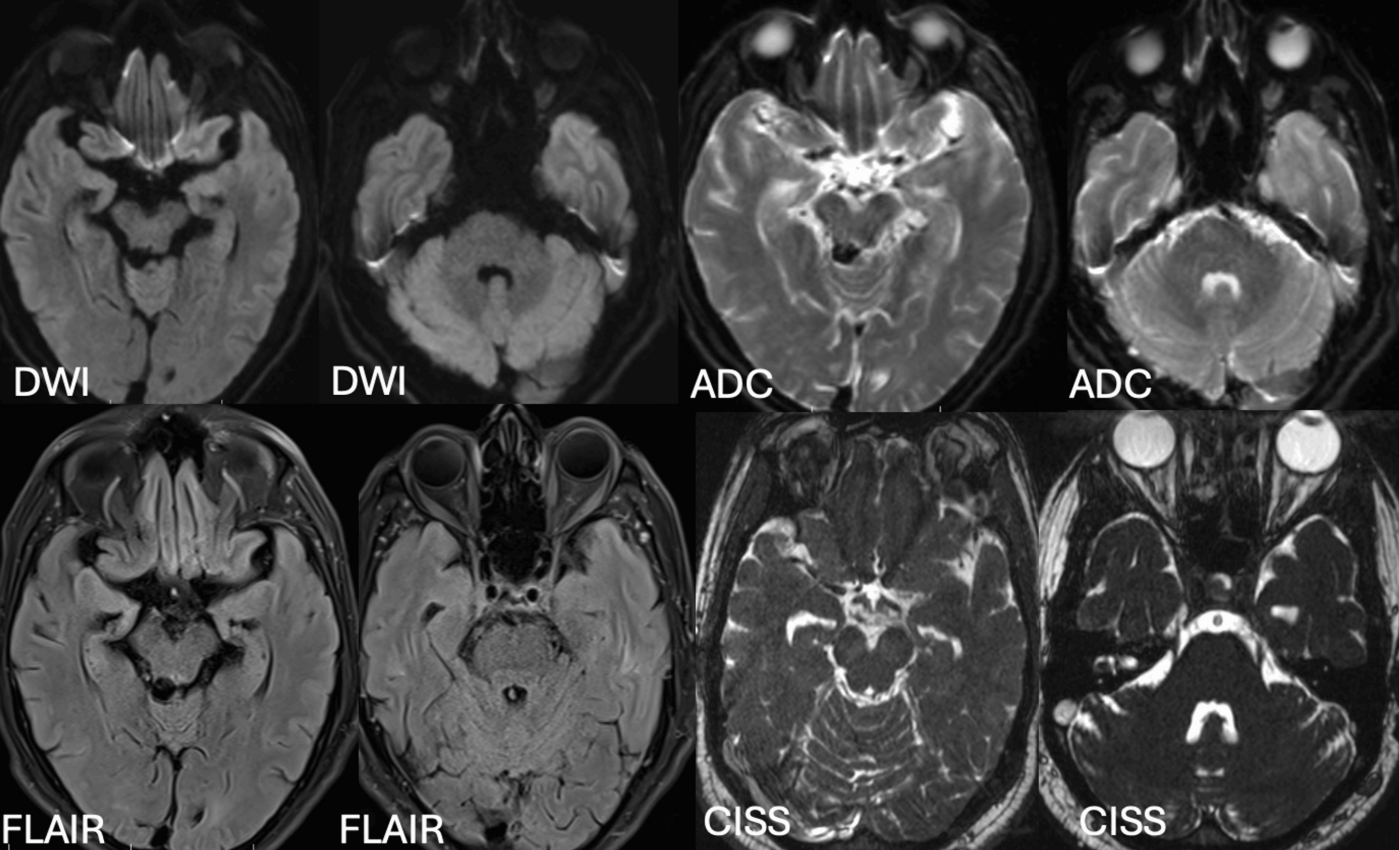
MRI brain plain and CISS sequence within normal limits DWI: diffusion-weighted imaging; ADC: apparent diffusion coefficient; FLAIR: fluid-attenuated inversion recovery; CISS: constructive interference in steady state
